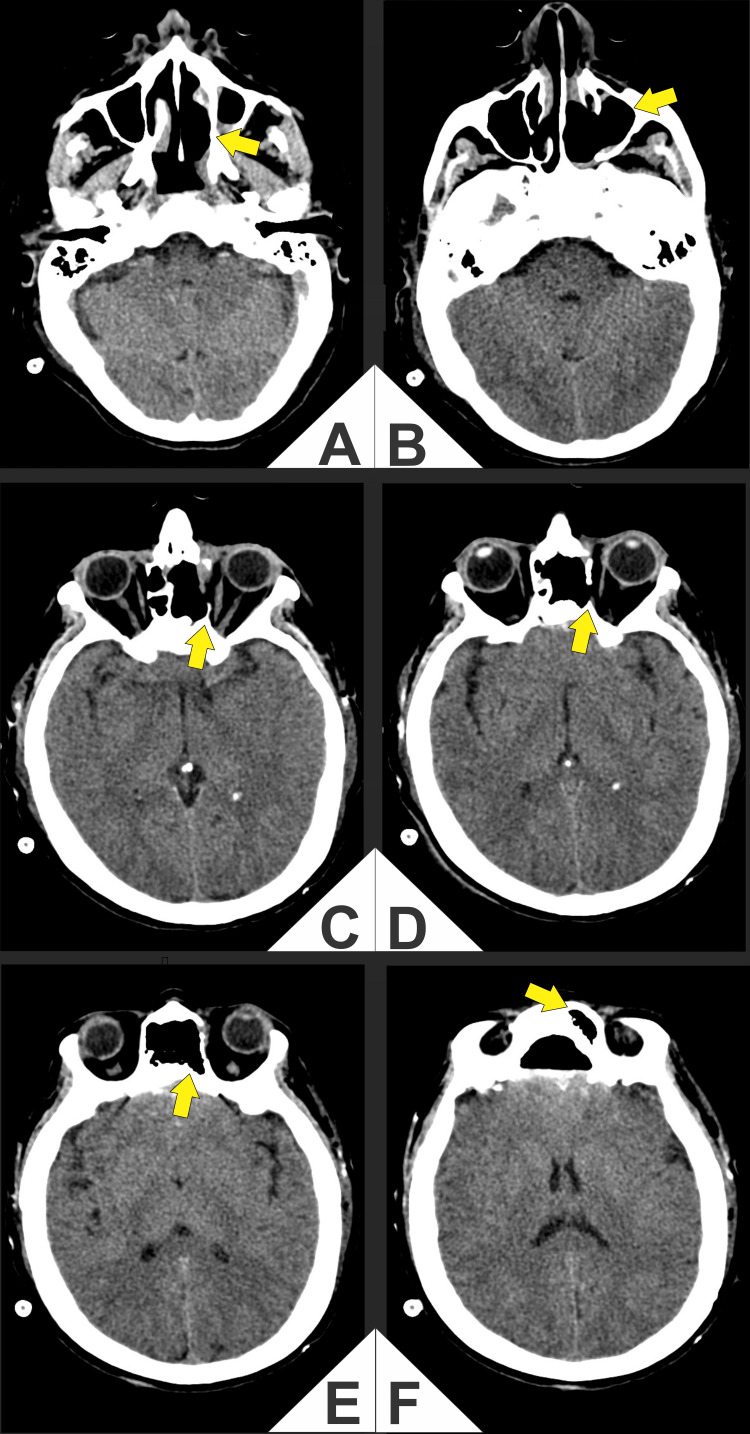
(A-F) Early postoperative axial computed tomography (CT). Arrows indicate the previous tumor bed in the left nasal cavity/ethmoid and medial orbital wall. Postoperative changes after bifrontal craniectomy and endoscope-assisted transcranial resection include anterior skull base reconstruction (titanium mesh with polymethylmethacrylate cranioplasty) and decompression of the medial rectus with reconstitution of the medial extraconal fat plane, with no evident residual mass. Reactive sinonasal opacification and minimal pneumocephalus/subgaleal air are expected in the early postoperative period.
